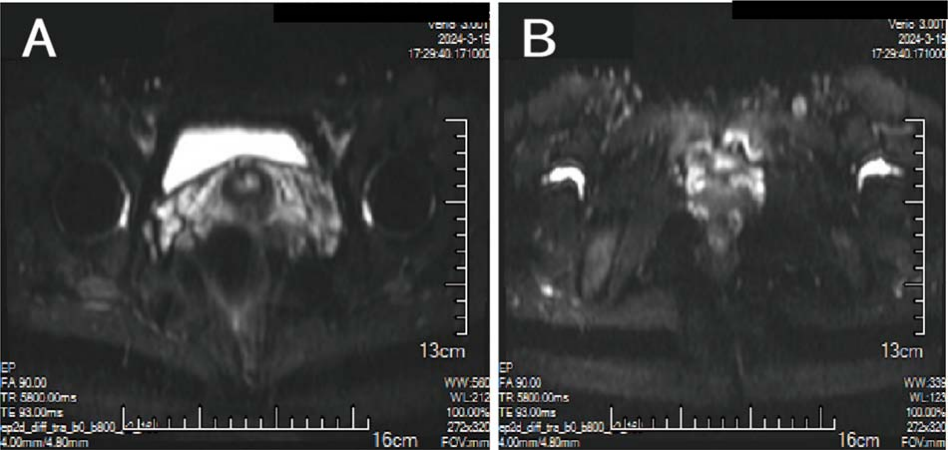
MRI: (A, B) A cluster of low/high mixed signals such as T1 and T2 can be seen on the left side of the anus, and the DWI signal is partially increased.
